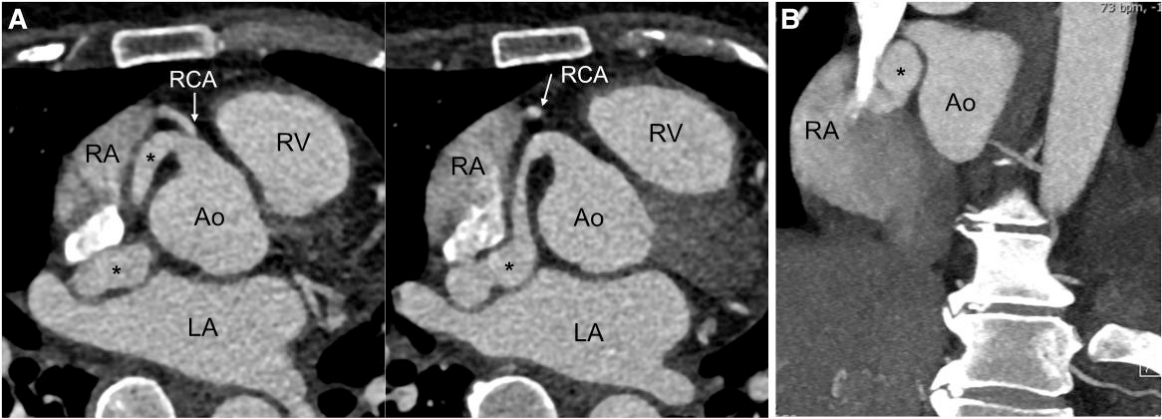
Computed tomography coronary angiography showed a coronary artery fistula from the RCA to the right atrium. (*A*) Axial oblique slices on computed tomography, (*B*) coronal view showing coronary artery fistula (asterisk) draining into the right atrium. RA, right atrium; RV, right ventricle; Ao, aortic root; LA, left atrium; RCA, right coronary artery.
